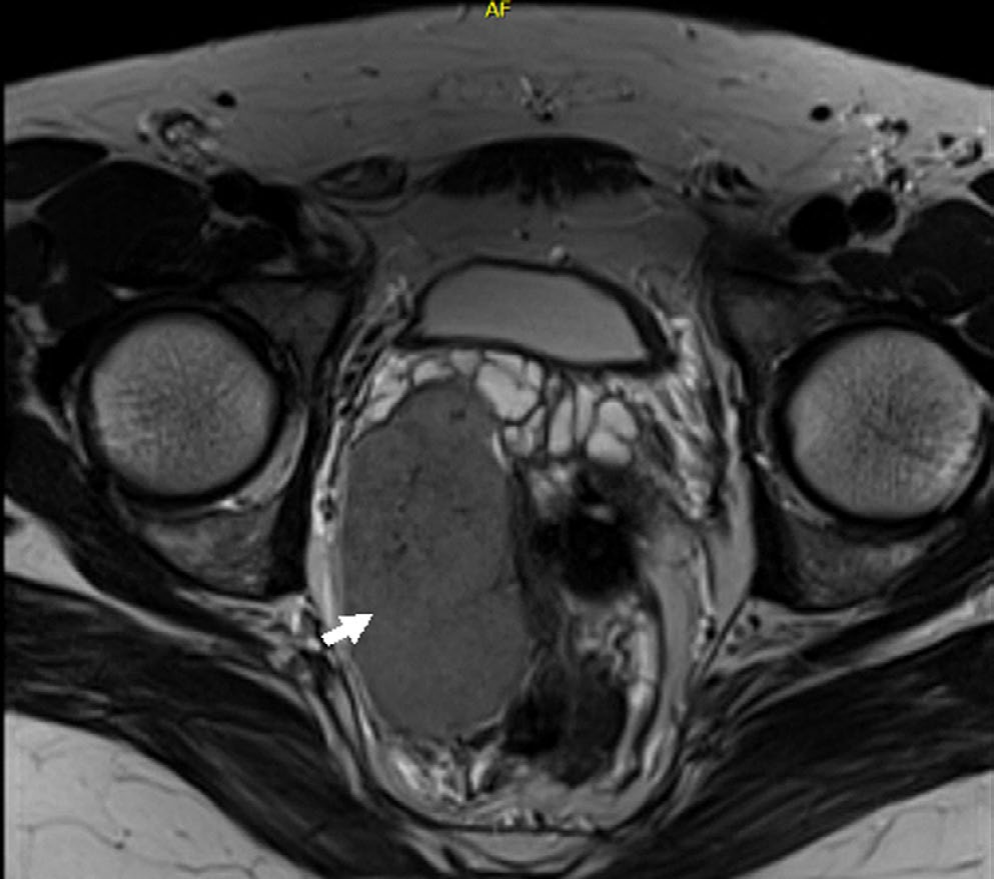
Axial view of a magnetic resonance imaging (MRI) of the pelvis, T2‐weighted sequence, showing a large pelvic well‐defined mass measuring around 12 by 10 cm, causing compression on the right seminal vesicle, and causing mild compression and deviation on the rectum without definite invasion
